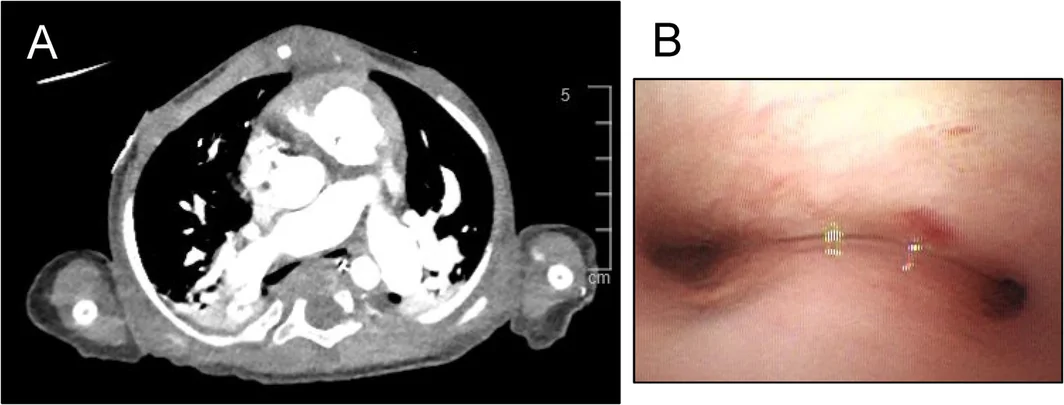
Imaging findings demonstrating tracheal bifurcation compression. (A) Contrast‐enhanced computed tomography showing narrowing of the tracheal bifurcation caused by extrinsic compression from dilated pulmonary arteries. (B) A bronchoscopic image revealing stenosis at the carina.
